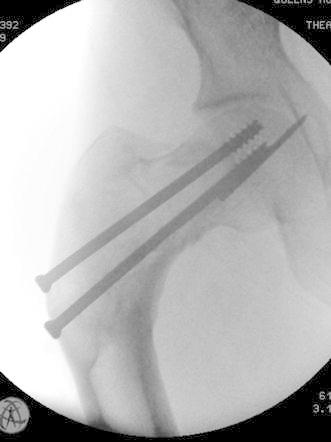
Antero-posterior radiograph of the right hip showing the broken guide wire during cannulated screw fixation of the fracture
